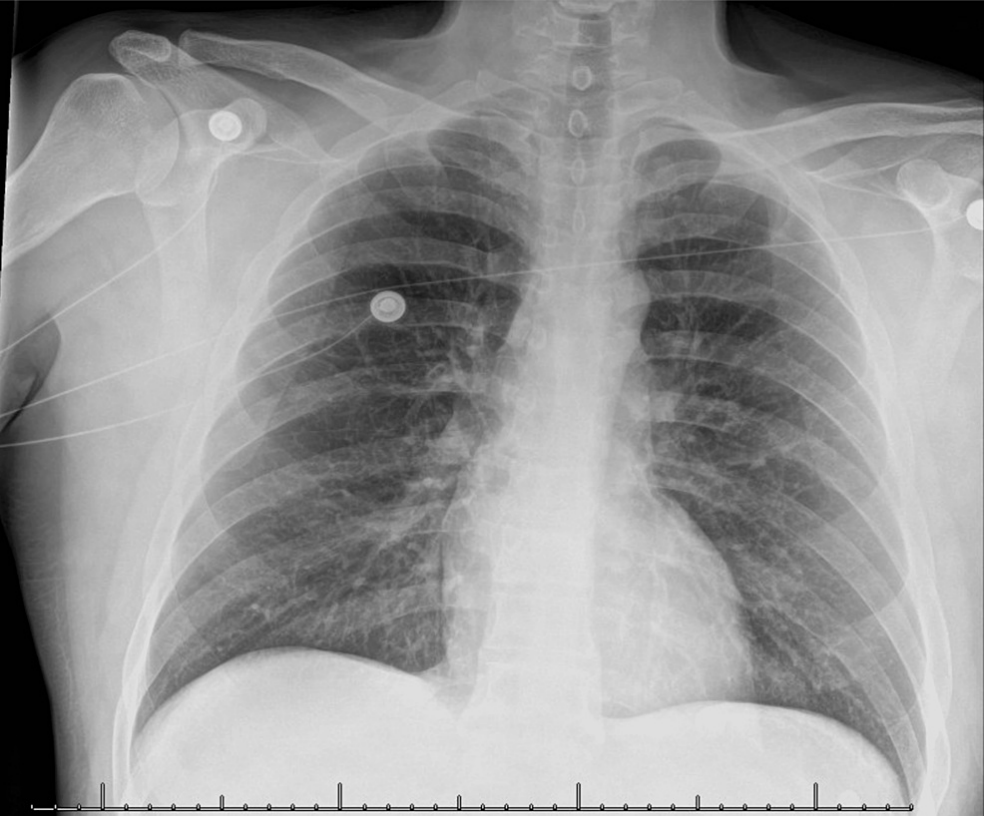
Chest X-ray. Negative chest x-ray showing no acute changes.
